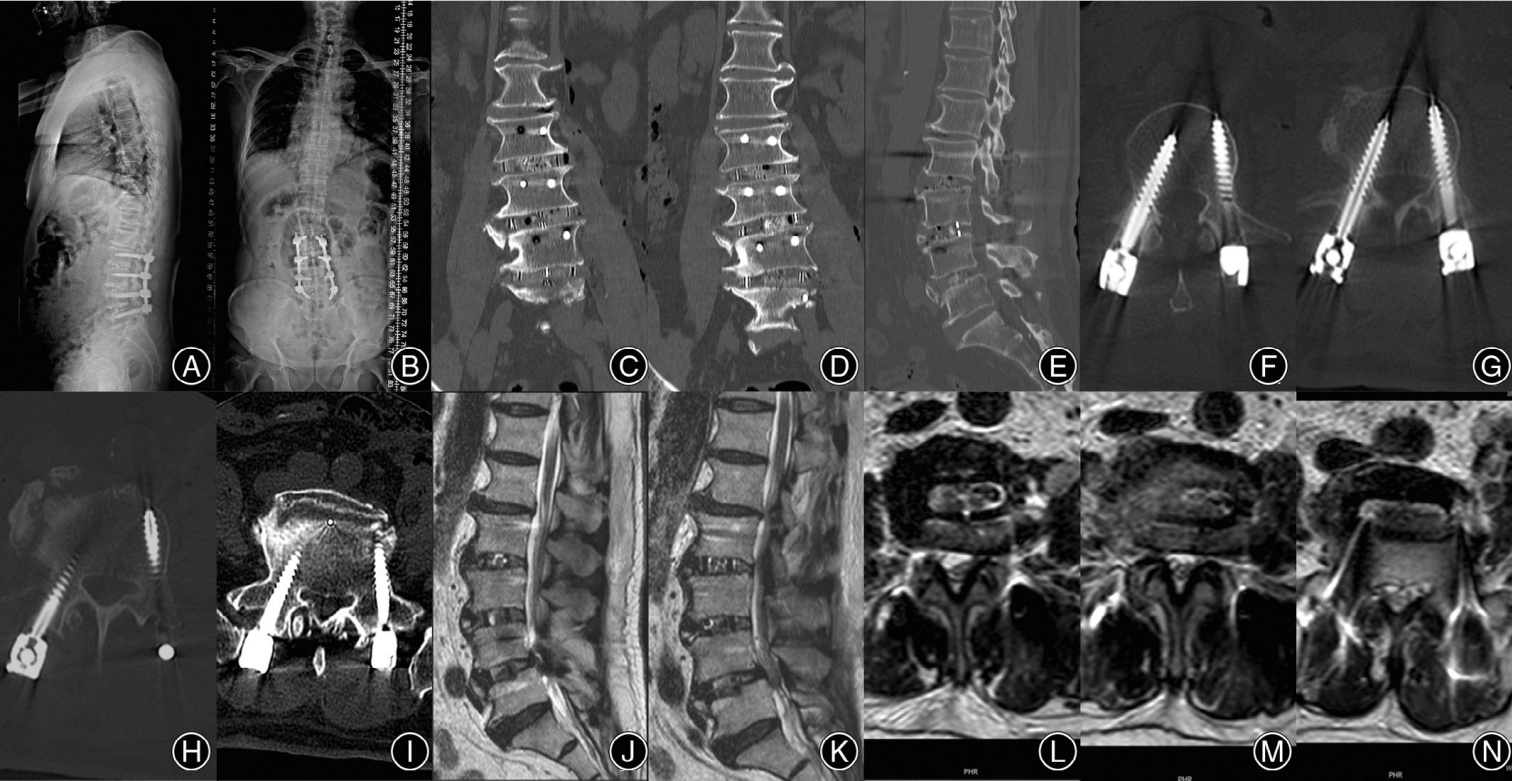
Coronal and sagittal imbalance of the lumbar spine were significantly improved on postoperative X‐ray (A, B). The osteophyte bridge at the contralateral disc space of L_3/4_ and L_4/5_ was released sufficiency (C–E). Postoperative CT showed that pedicles were all grade‐A according to the Gertzbein–Robbins classification and the degree of lumbar spondylolisthesis was significantly improved (F–I). Postoperative MRI showed that the degree of lumbar spinal stenosis was significantly improved, and the Schizas grades of L_3/4_ and L_4/5_ segments were A3 and A4, respectively (J–N).
